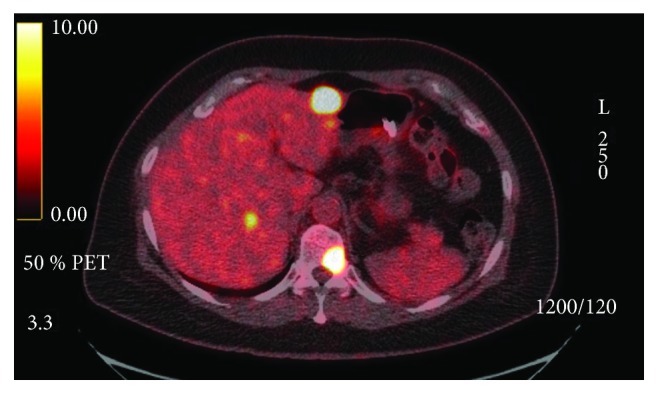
PET/CT image prior to immunotherapy.
